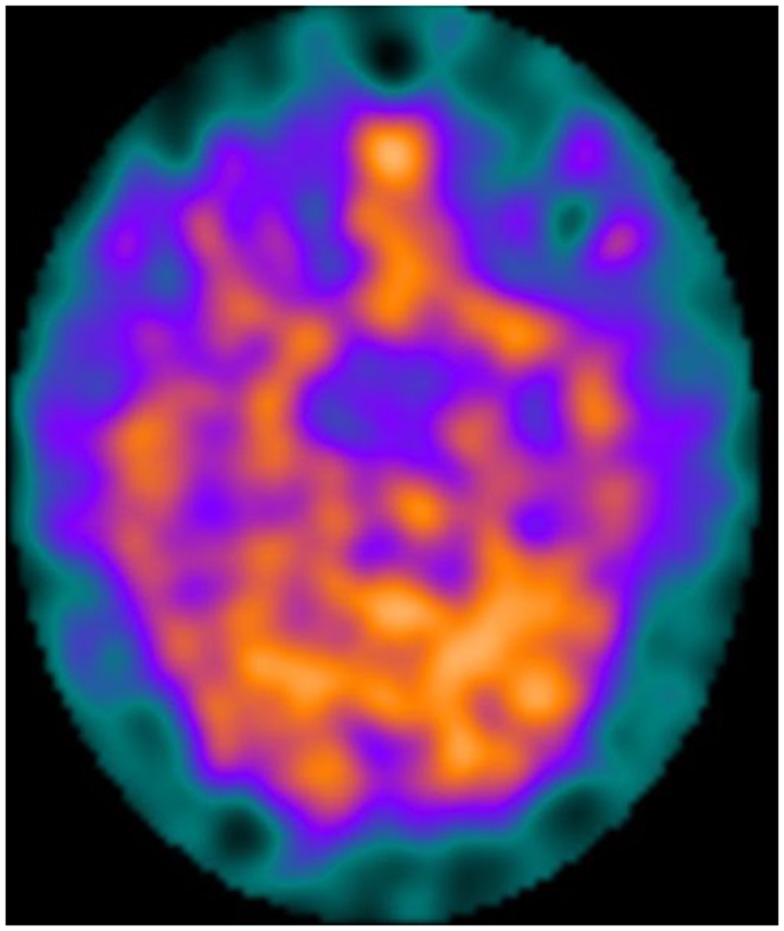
A female patient with active Kleine–Levin syndrome with a normal SPECT perfusion pattern.
